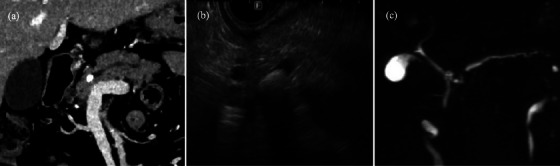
Contrast‐enhanced computed tomography (a), endoscopic ultrasonography (b), and magnetic resonance cholangiopancreatography (c) show a pancreatic stone in the main pancreatic duct and caudal pancreatic duct dilatation.
